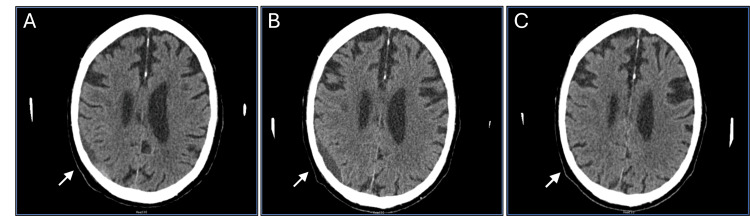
Follow-up CT scans show CSDH over time before and after TXA treatment (A & B) Follow-up CT scans show the progression in CSDH size and local mass effect over time before TXA treatment. (A) The size of the hematoma was 7 mm in June 2022, which indicates an increase in volume compared to the initial presentation. (B) The size of the hematoma was 11 mm in July 2022, which indicates an increase in the hematoma volume compared to the previous follow-up visit. (C) The CT scan shows complete resolution of the hematoma after the TXA treatment for one month. The previously noted subdural fluid collection in the right parietal area has completely resolved and the brain expanded in that area.
